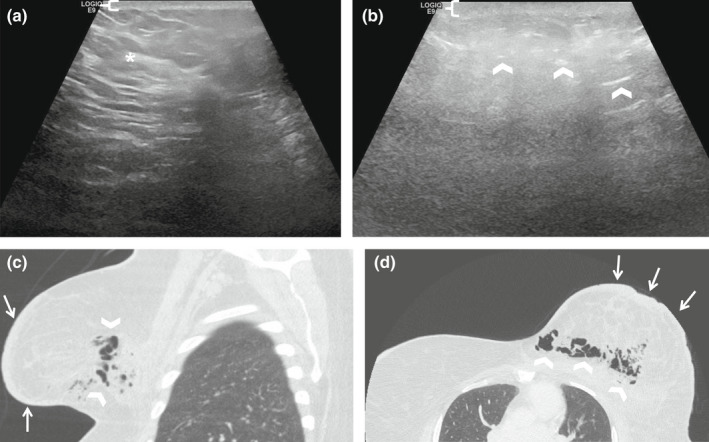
43‐year‐old woman with a history of diabetes and obesity presented with clinical signs of cellulitis and mastitis. Ultrasound was performed with a ML6‐15 MHz transducer with the frequency set to 11 MHz, and the images were acquired from the skin to the chest wall. Targeted ultrasound imaging of the area of clinical concern in the left breast demonstrated mild skin thickening measuring 3 mm (bracket) and mild echogenic breast parenchyma (asterisk) involving the lateral portion of the breast (a). Additional imaging at the area of marked erythema in the medial and inferior breast showed skin thickening measuring 4 mm (bracket) and multiple scattered echogenic foci (chevrons) with posterior dirty shadowing suggestive of subcutaneous air (b). The ultrasound artefact obscured the visualisation of the breast parenchyma posteriorly. Chest CT was immediately performed, revealing gas in the left breast (chevrons) with overlying skin thickening (arrows) as seen on a sagittal image on lung windows (c). Axial chest CT image on lung windows demonstrates air throughout the inner and central left breast extending into the left parasternal subcutaneous tissue (chevrons) with skin thickening (arrows) (d). The on‐call breast surgeon was notified of these critical findings, and the patient was subsequently taken for wide local debridement in the operating room the same day. She required multiple subsequent surgical debridements and ultimately had a mastectomy.
